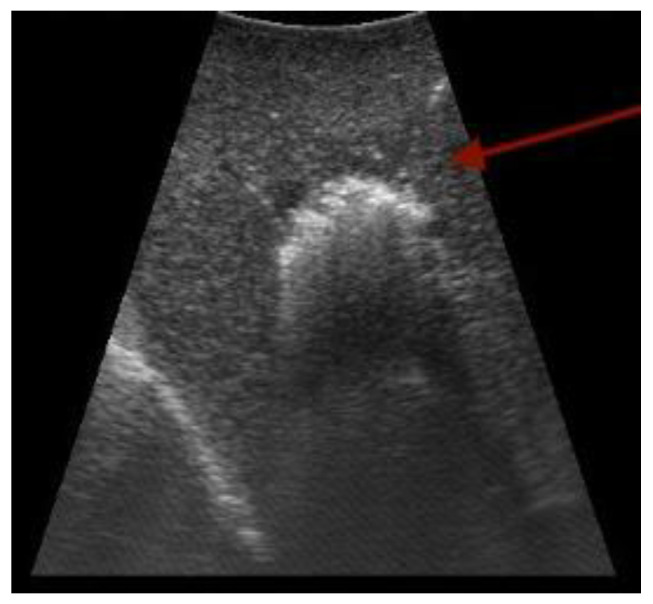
Ultrasound-guided radiofrequency ablation in the liver (arrow points toward the needle). Original figure.
